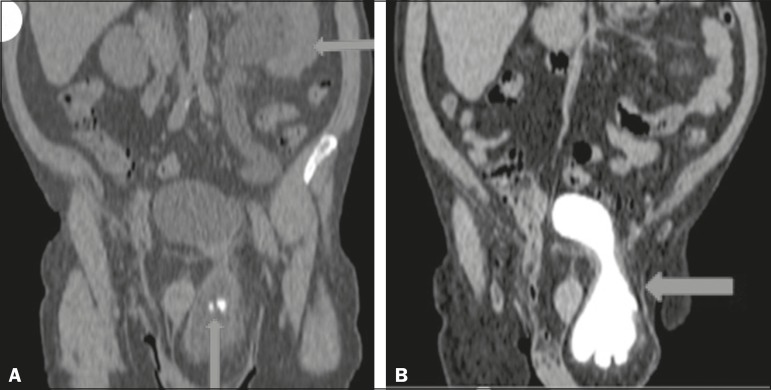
A: CT with coronal reconstruction showing a left-sided
